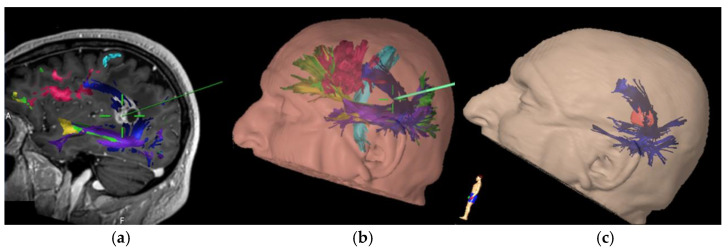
Intraoperative screenshots with navigated tractography of left temporo-parietal high-grade glioma involving the middle part of AF (a) T1 contrast enhanced MRI; (b) 3d volume rendering with coloured tracts; (c) 3d volume rendering with AF and tumor.
